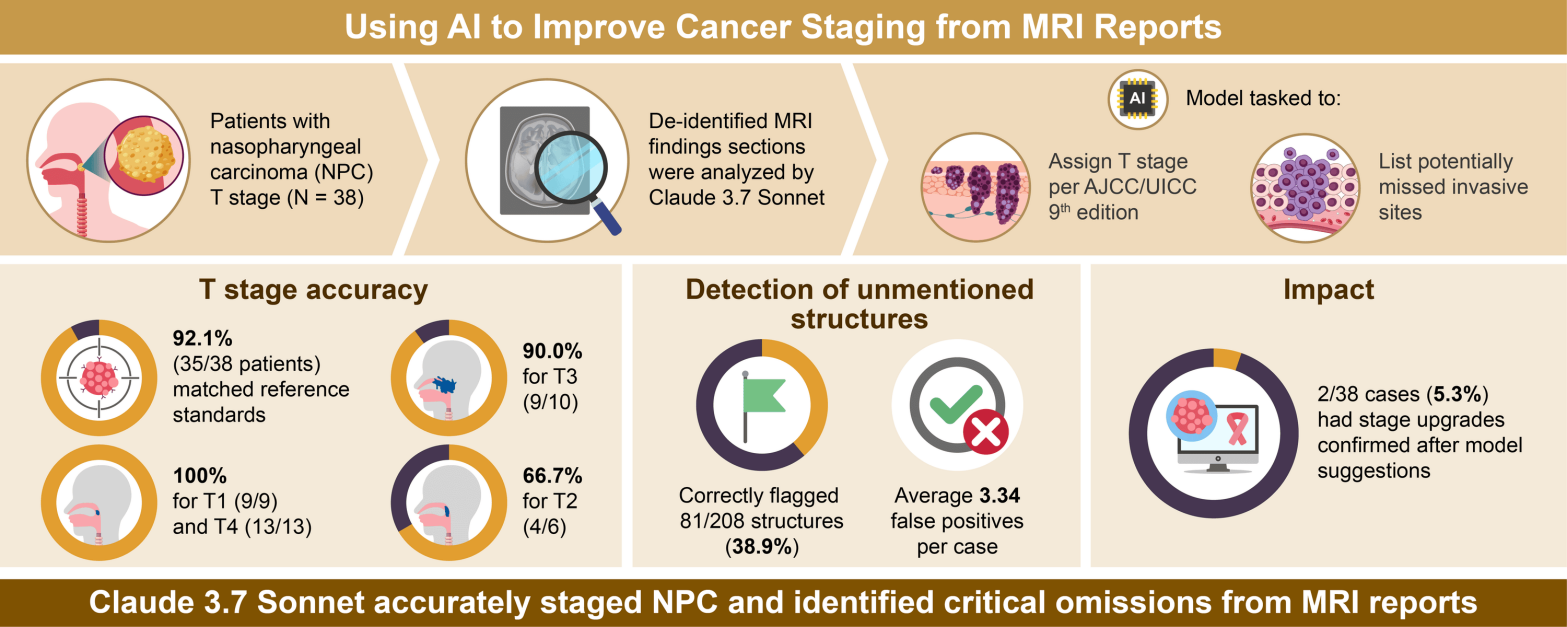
Overview of the study NPC: nasopharyngeal carcinoma; AJCC/UICC: American Joint Committee on Cancer/Union for International Cancer Control; AI: artificial intelligence; MRI: magnetic resonance imaging Image Credit: Authors
